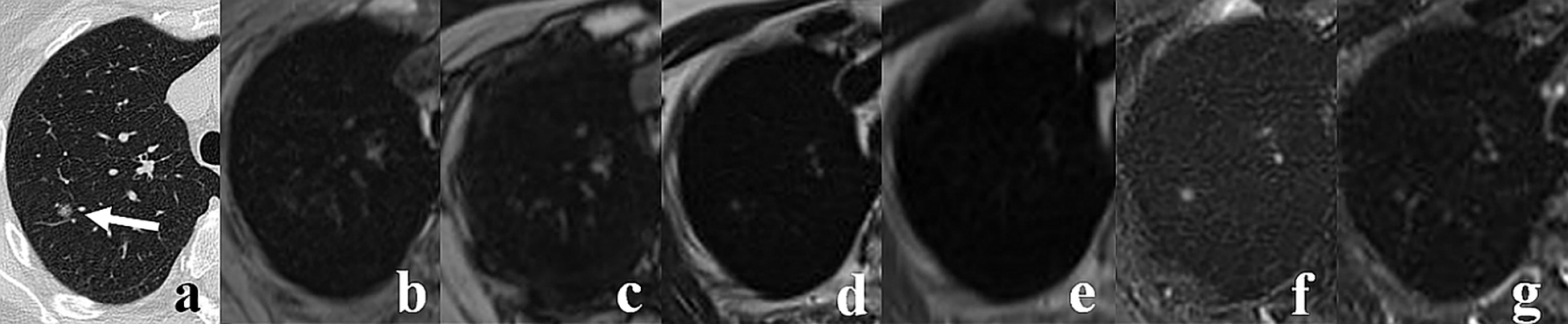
A 71-year-old woman with 7 mm pulmonary nodule in the right upper lobe (arrow). The nodule is demonstrated on CT (A) and on most of T2-weighted sequences, including: T2 TSE (D), T2 SPIR (F) and T2 STIR (G). The readers did not identify this lesion on T1 VIBE (B), T1 out-of-phase (C) and T2 HASTE (E) images.
